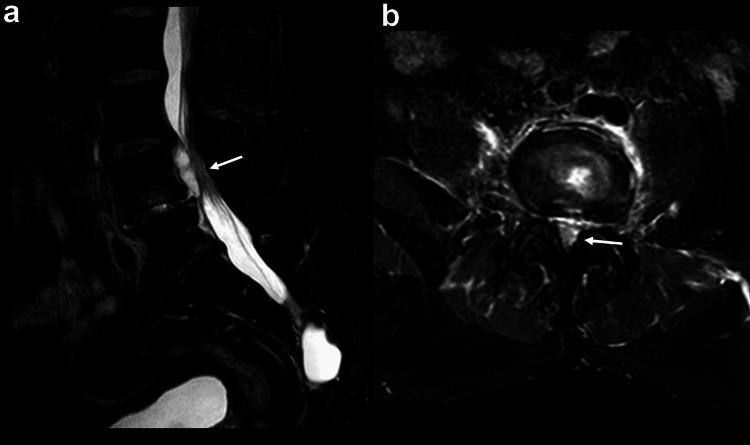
Sagittal (a) and axial (b) fat-suppressed T2-weighted MRI Severe canal stenosis at the level of L4 due to the collection, with an anteroposterior diameter of the dural sac measured at 4 mm and loss of the visibility of the rootlets and cerebrospinal fluid. MRI, magnetic resonance imaging
